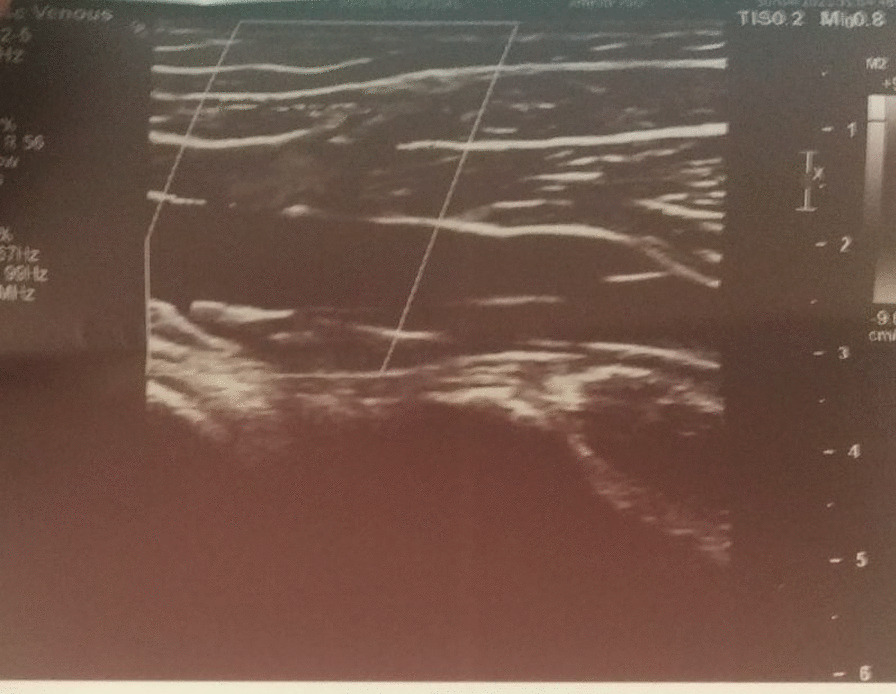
Doppler of proximal end of Superficial Saphenous Vein
